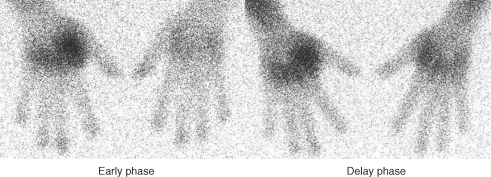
Radiographs of the right hand show an increase in soft tissue shadow in the first web space.
